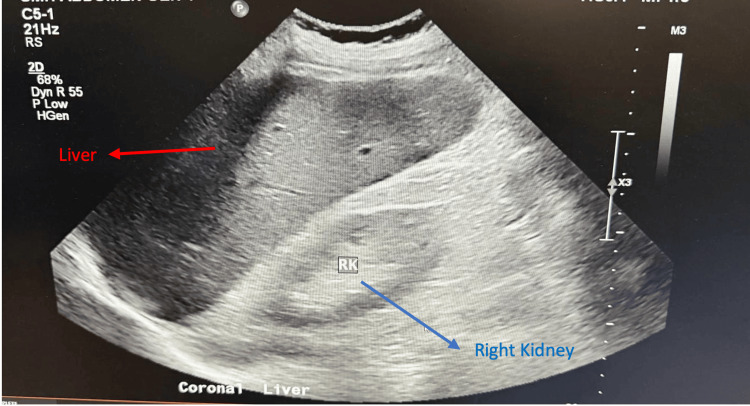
Ultrasound abdomen showing mild hepatosplenomegaly with no evidence of bile obstruction.
